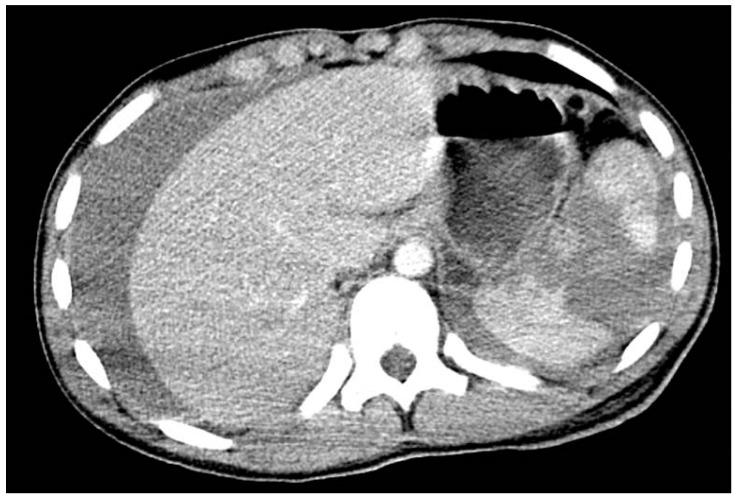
CT with IV contrast shows free fluid around the liver and spleen (hemoperitoneum) with complete disruption of the spleen parenchyma in terms of rupture.
